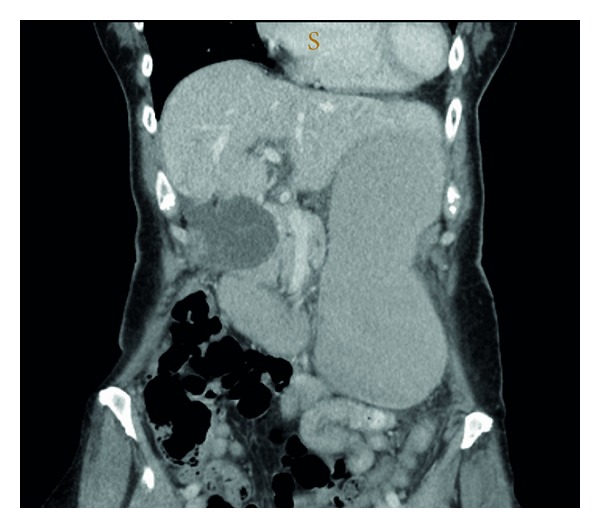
Reconstructed coronal images on computed tomography demonstrating the dilated and relatively inferiorly placed gallbladder. Acute gastric distension was also present due to duodenal compression by the very dilated gallbladder (not shown).
